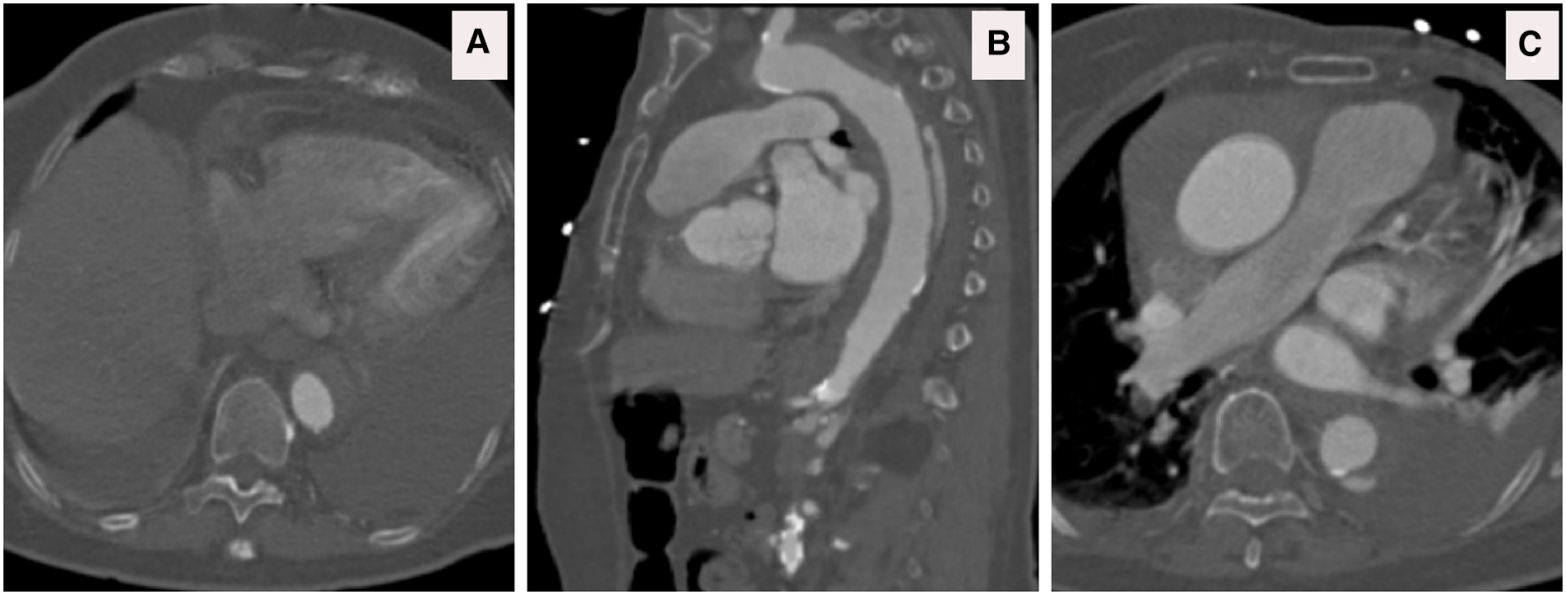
(A) CTA done at one-hour post-collapse in another institute shows acute aortic hematoma with interscapular pain during the COVID-19 pandemic lockdown. (B) CTA sagittal view done at 20 h post-collapse in another institute confirmed a small bleed into the acute mural hematoma. (C) CTA axial views demonstrated that the hematoma extends proximally and distally.
